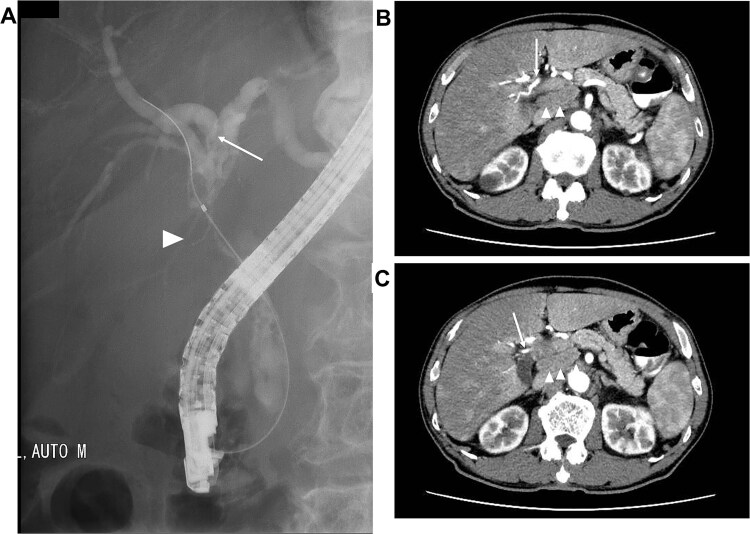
Images before neoadjuvant GCD immunochemotherapy (A) ERC revealing biliary stricture extending from the CHD (arrow head) to the hepatic hilum. Right posterior branch (arrow) joins the left hepatic duct. (B and C) dynamic CT demonstrating the tumor invades the RHA (arrow), and the lymph node surrounding the common hepatic artery and retropancreatic area is swollen (arrow head).
